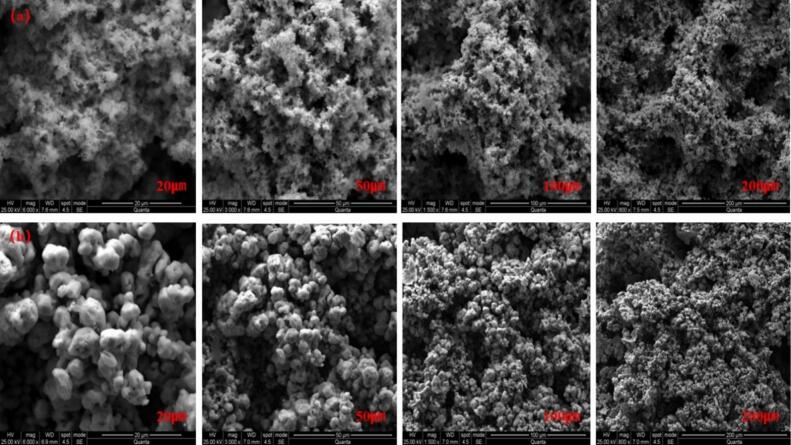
SEM of the deposited layer. a. Conventional electrodeposition; b. Ultrasound-assisted electrodeposition; Deposition potential: −0.80 V, Deposition time: 30 min, Temperature 25℃, ultrasonic power 100 W.
